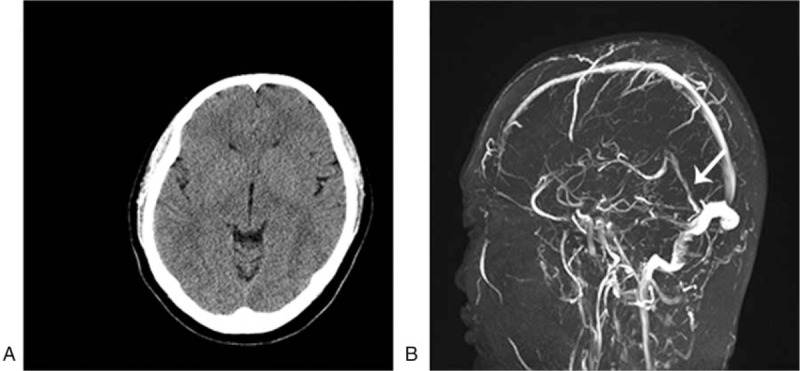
(A) Noncontrast cerebral CT scan showing the original dense clot sign of straight sinus has disappeared. (B) Straight sinus is seen on cerebral MRV (white arrow). CT = computed tomography, MRV = magnetic resonance venography.
